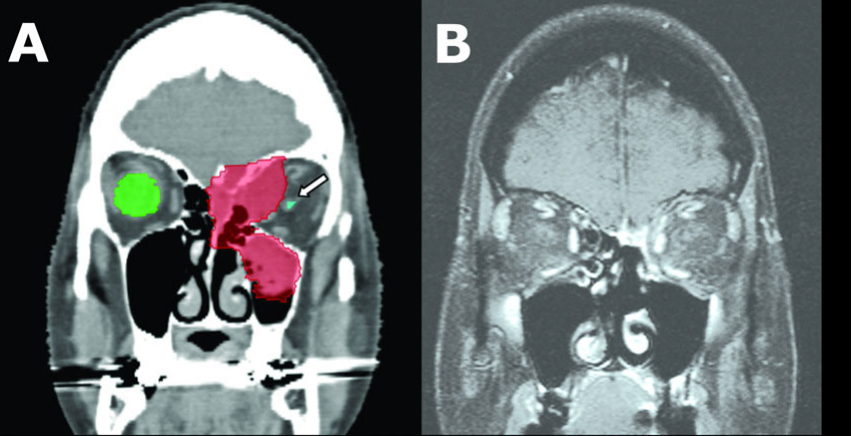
57-year-old woman with squamous cell carcinoma of the left ethmoid sinus, orbit and anterior skull base. Prior to consideration of radiosurgery, the original treatment plan was craniofacial resection with left orbital exenteration. She was treated with 3000 cGy in 5 stages. (A) Coronal CT with contrast prior to radiosurgery with treatment-planning contour. The tumor is shaded in red. Note proximity of left optic nerve. *White arrow: optic nerve*. (B) Coronal MRI with contrast 13 months after radiosurgery showing dramatic response. Currently, the patient continues to have normal binocular vision nearly 4 years after treatment.
